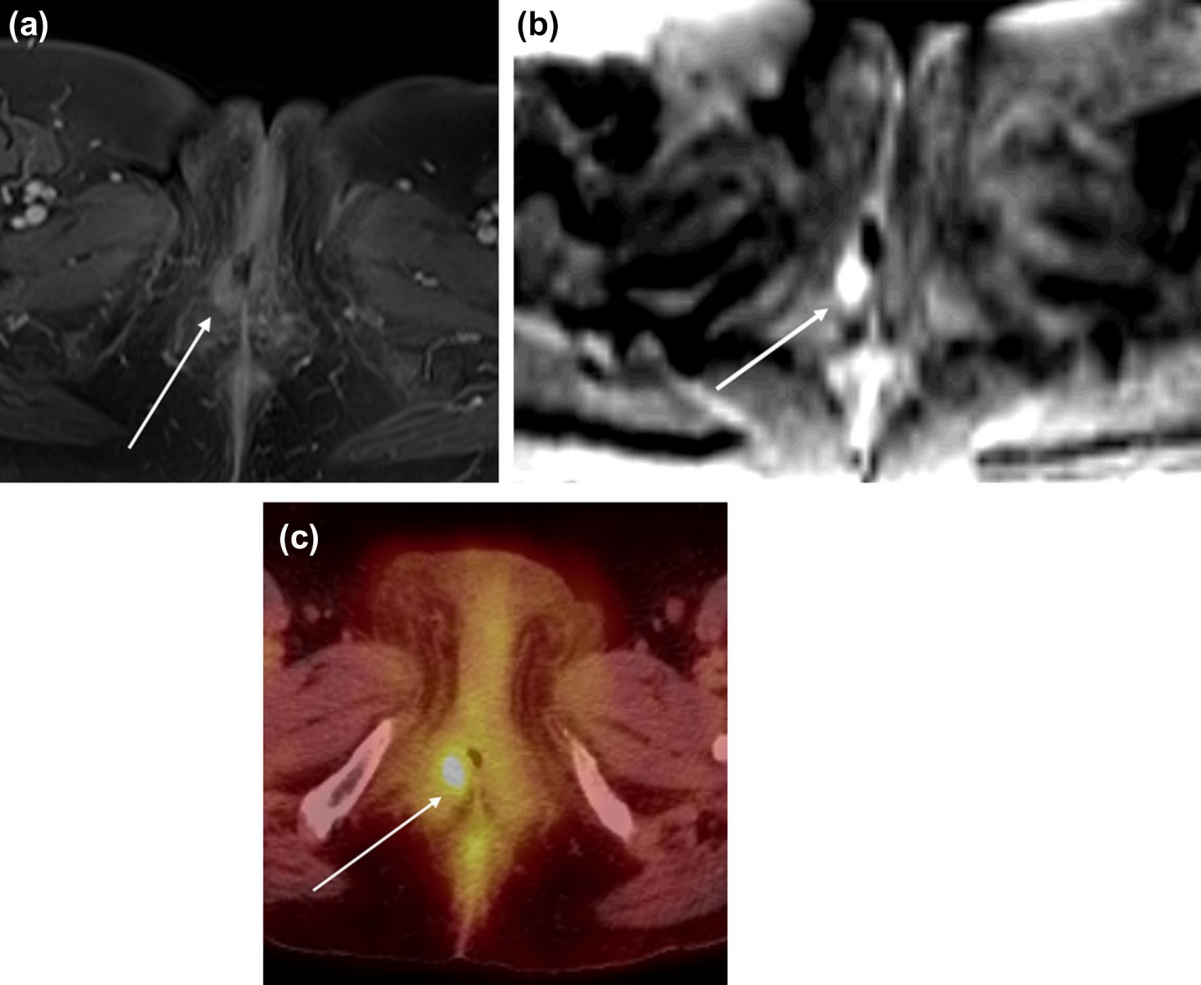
56-year-old woman with FIGO stage 4A SCC of the vulva status post-hysterectomy, bilateral oophorectomy, and radiation with recurrent disease 6 months later. a Axial T1-weighted contrast-enhanced and b DWI (b = 1000) MR images show a nodule measuring around 1 cm in the right vulva with enhancement and restricted diffusion (arrow). ADC image shows corresponding hypointensity (not shown). c Axial PET-CT image demonstrates soft tissue thickening in the right vulva with focal intense FDG uptake (arrow)
